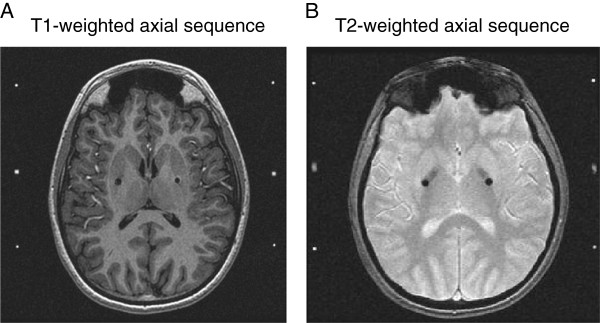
Postoperative magnetic resonance images for the targets of bilateral Globus Pallidus Pars Interna from a representative patient. (A) T1-weighted and (B) T2-weighted axial sequences are shown with the target highlighted with black dots.
